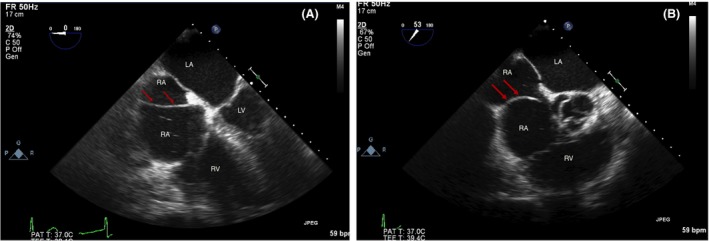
Transesophageal echocardiogram (A and B). Red arrows indicating a membrane dividing RA into two chambers. LA, left atrium; RA, right atrium; LV, left ventricle; RV, right ventricle.
